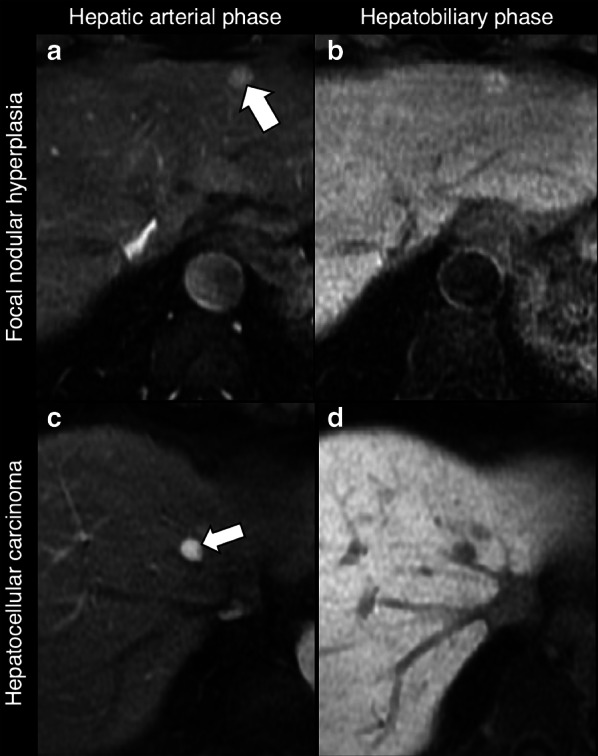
Differential diagnosis of hypervascular lesions in the arterial phase in oncologic patients. Top row: 53-year-old woman with breast cancer and focal nodular hyperplasia. Gadobenate dimeglumine-enhanced MRI demonstrates a focal nodular hyperplasia (arrow) that (a) enhances in the hepatic arterial phase and (b) is hyperintense in the hepatobiliary phase. Bottom row: 58-year-old man with pharyngeal carcinoma and hepatocellular carcinoma. Gadoxetate disodium-enhanced MRI demonstrates a small HCC nodule (arrow) that (c) enhances in the hepatic arterial phase and (d) is hypointense in the hepatobiliary phase
